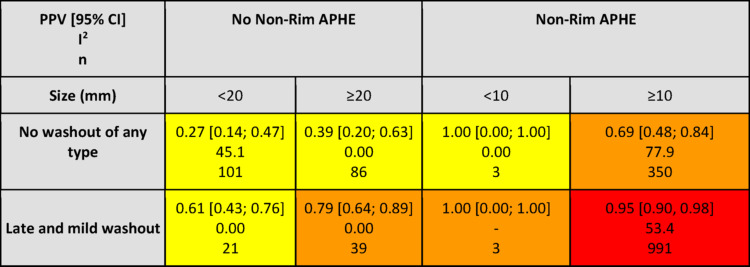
Diagnostic table shows the random-effects model of positive predictive values (PPVs) for diagnosis of hepatocellular carcinoma (HCC) for contrast-enhanced ultrasound (CEUS) Liver Imaging Reporting and Data Systems (LI-RADS) major feature combinations. The pooled PPV estimates and 95% CIs for each LI-RADS category were as follows: LR-3, 40.4% (95% CI: 27.2–55.1); LR-4, 69.7% (95% CI: 49.7–84.3); LR-5, 95.1% (95% CI: 90.2–97.6). Each cell in the diagnostic table shows PPVs at the top and I^2^ values in the middle, which indicate diagnostic performance and between-study heterogeneity, respectively. Each cell also shows the sample size (n) at the bottom. Data in parentheses are 95% CIs. PPVs increased with observation size (< 10 mm to ≥ 10 mm), presence of arterial phase hyperenhancement (APHE), and the presence of late and mild washout. LR-3 combinations (yellow) showed the lowest PPVs and often low heterogeneity (I^2^), LR-4 combinations (orange) showed intermediate PPVs with variable heterogeneity, whereas LR-5 combinations (red) demonstrated consistently high PPVs with generally high I^2^ values
